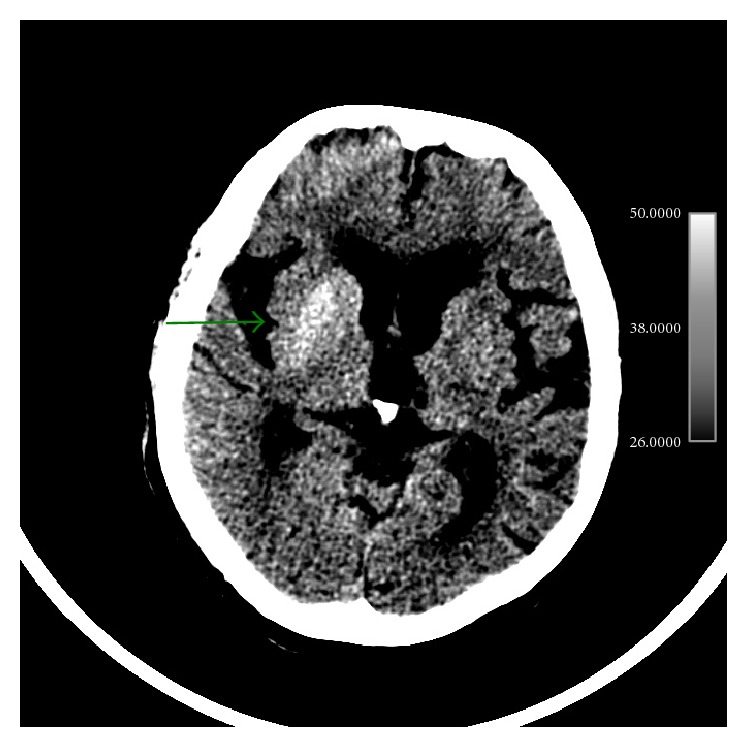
Findings: unenhanced axial brain CT depicts hyperdense striatum (caudate nucleus and putamen). This finding is better seen using narrow window width and higher center settings.
